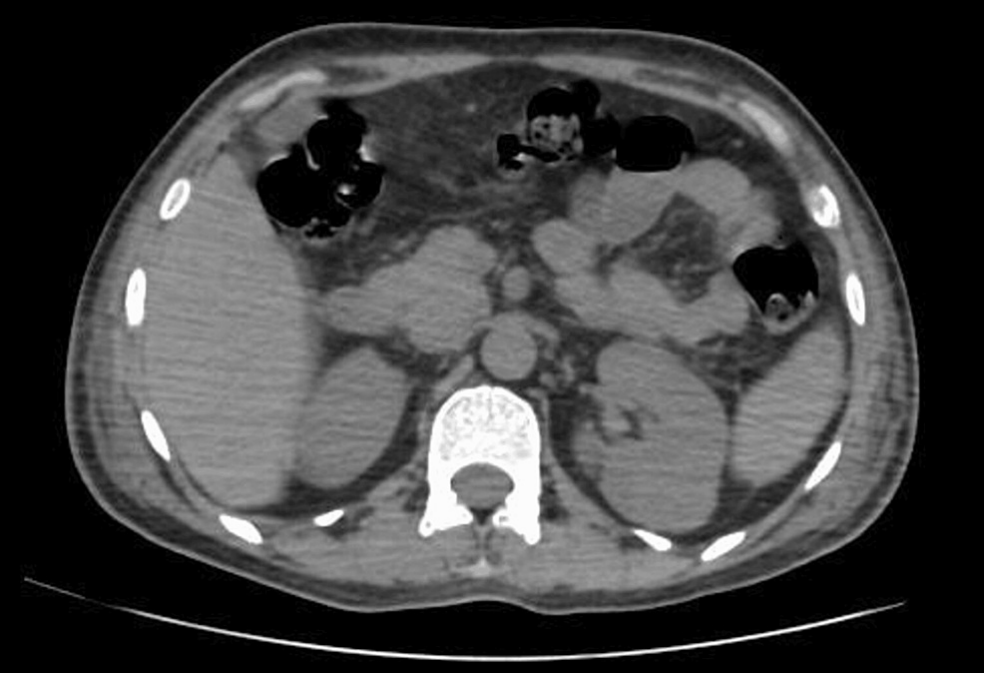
Contrast-enhanced computed tomography of the whole abdomen
